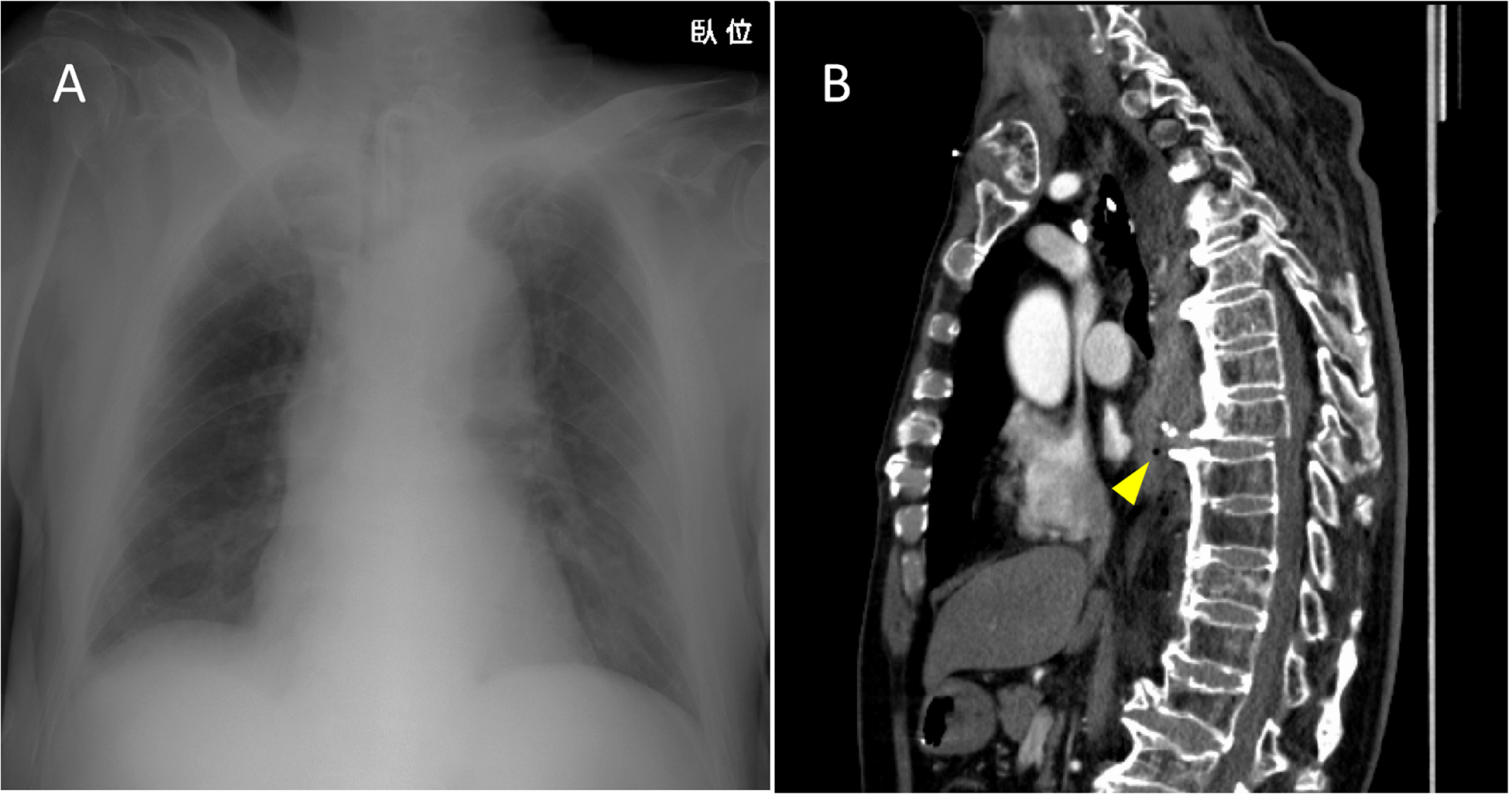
Images from Case 1. a Chest radiography shows extended opacity of the right chest, and b computed tomography shows a T8-burst fracture (arrow head)
